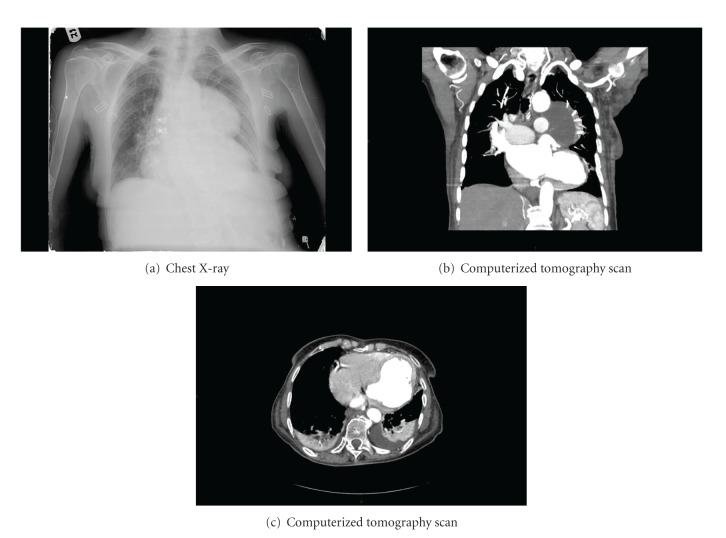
(a) A posterior-anterior X-ray of the chest which reveals a space-occupying lesion with clearly defined borders. The lesion is of single consistency without calcification and merges with the lower border of the heart situated at a wide angle to the shadow of the heart, appearing as a mass in the anterior middle mediastinum. (b) Computerized tomography scan reveals a cystic process in the anterior middle mediastinale region with a double layer of fluid, one of which is consistent with blood. The process merges with the left ventricle. (c) CT Frame 205 of the same exam revealed thinning of the posterior lateral wall of the left ventricle with an aneurysm of approximately 50 millimeters, expanding from the left ventricle.
